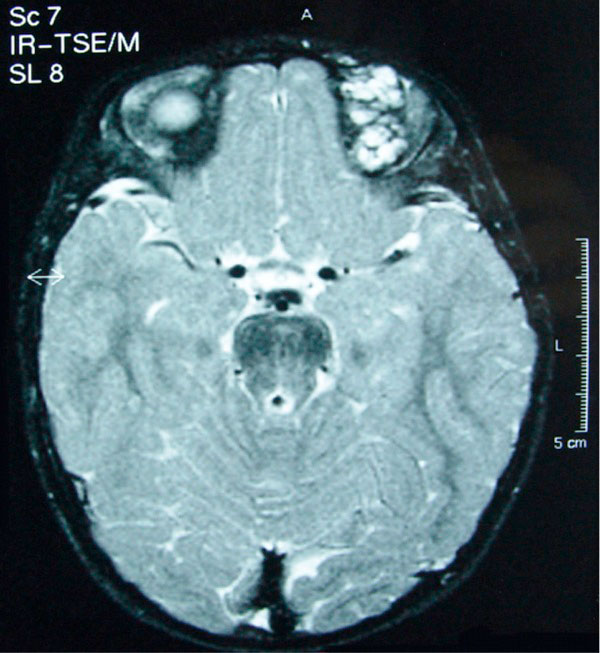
Cavernous hemangioma of the left upper eyelid in the same patient, on an axial T2- weighted MRI, after intravenous injection of Gadolinium.
